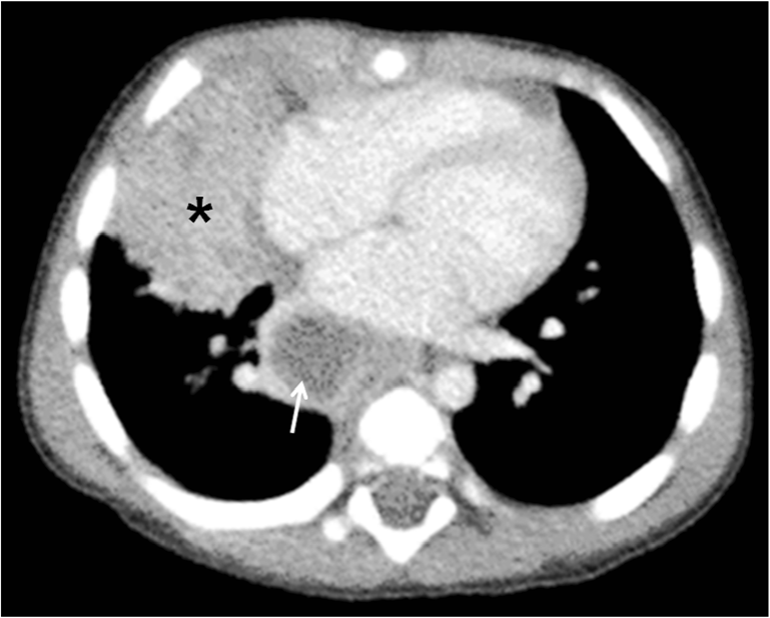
Primary progressive tuberculous disease in a 15-month-old boy. Axial contrast-enhanced CT image shows progressive Ghon focus (*asterisk*) in the right middle lobe and progressive hilar lymphadenopathy (*arrow*). The enlarged lymph node demonstrates the characteristic central necrosis with peripheral enhancement (rim sign)
